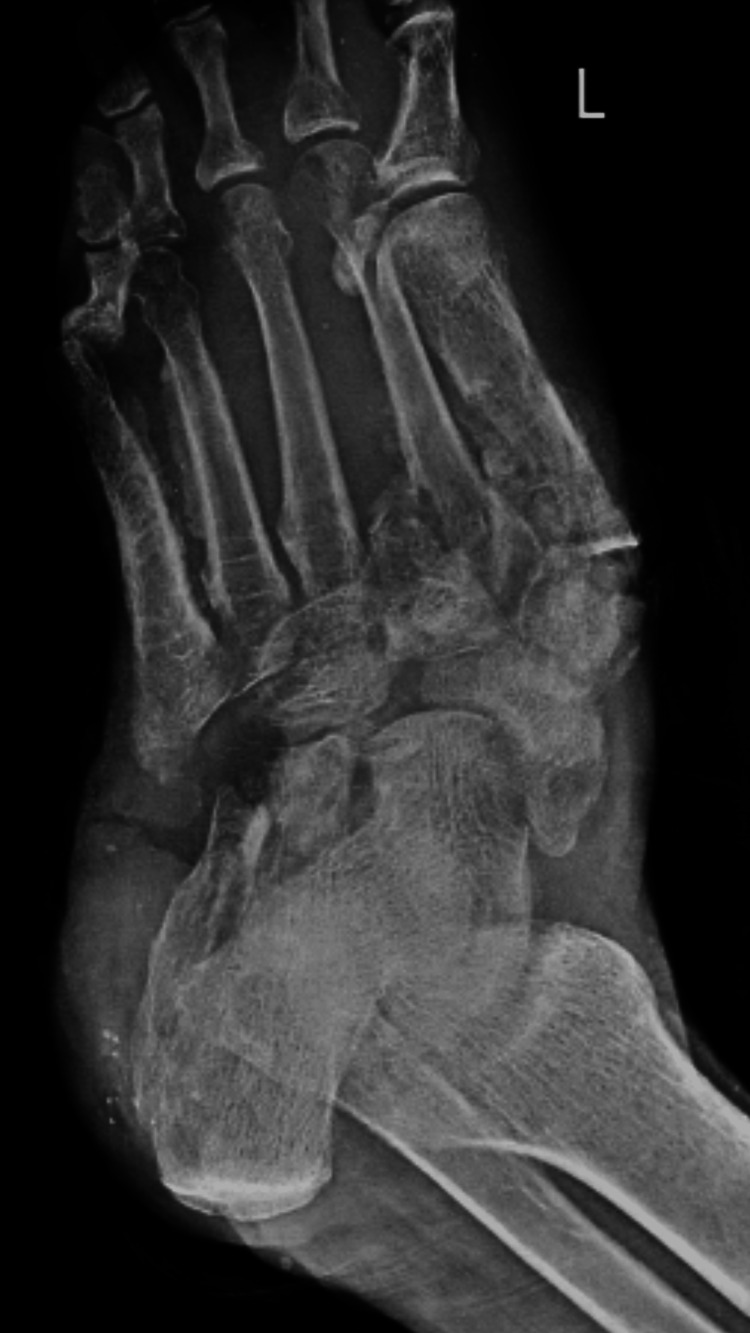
Preoperative X-ray of the ankle and toes X-ray of the ankle and toes shows a Lisfranc fracture of the left foot and all cuneiform and cuboid fractures of the left foot
